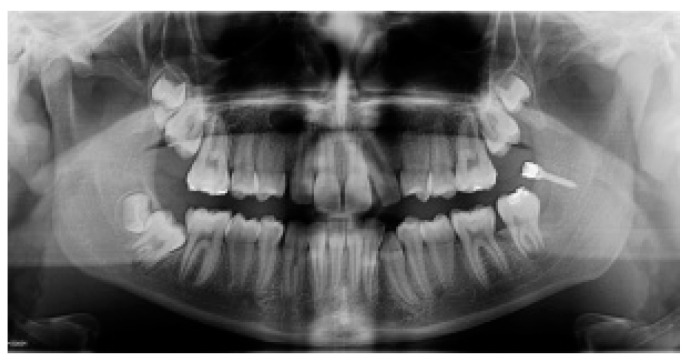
Post-treatment panoramic radiograph showing the correct inclination of 3.7 before miniscrew removal.
